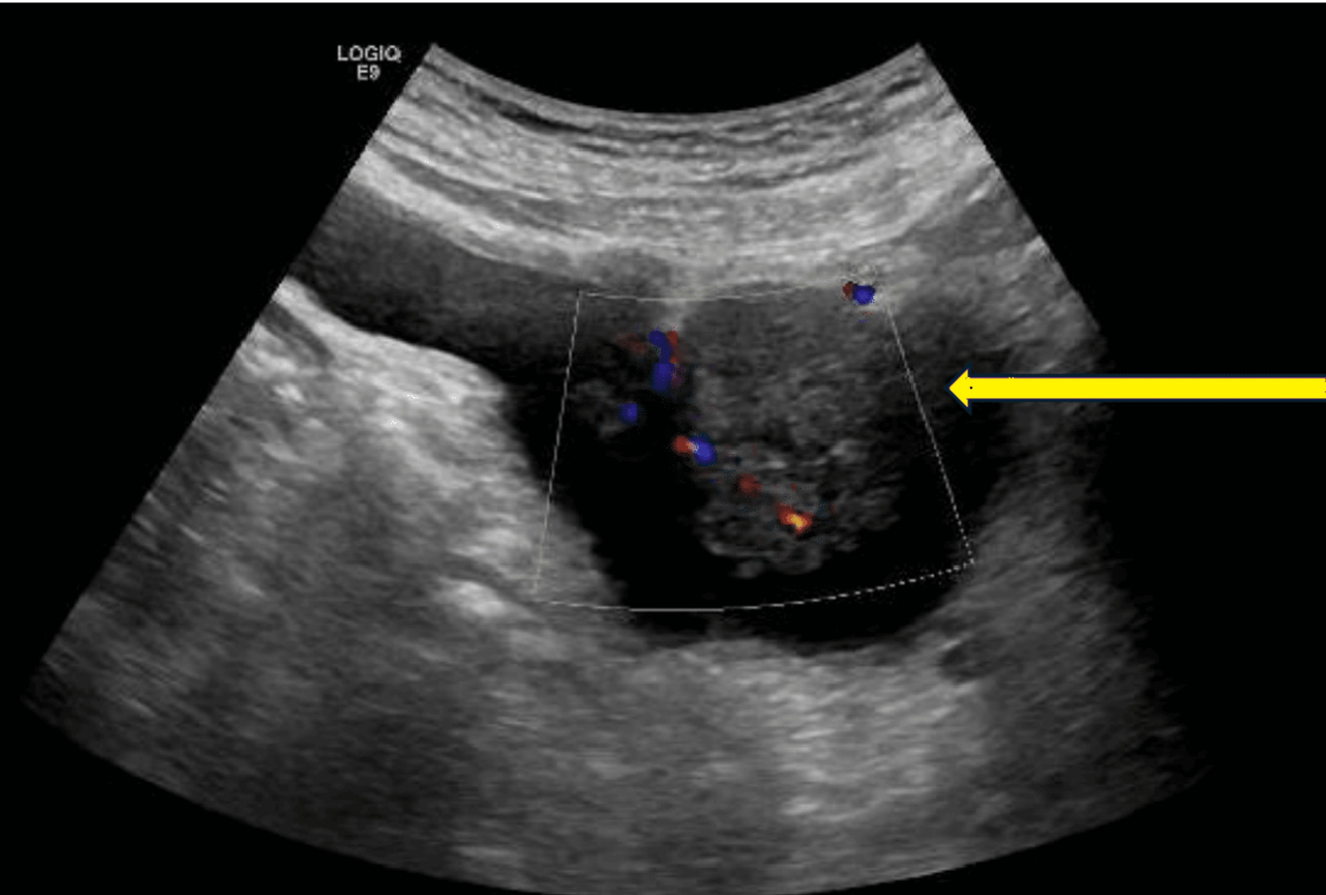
Ultrasound scan with Doppler views showing vascularity to the lesion
